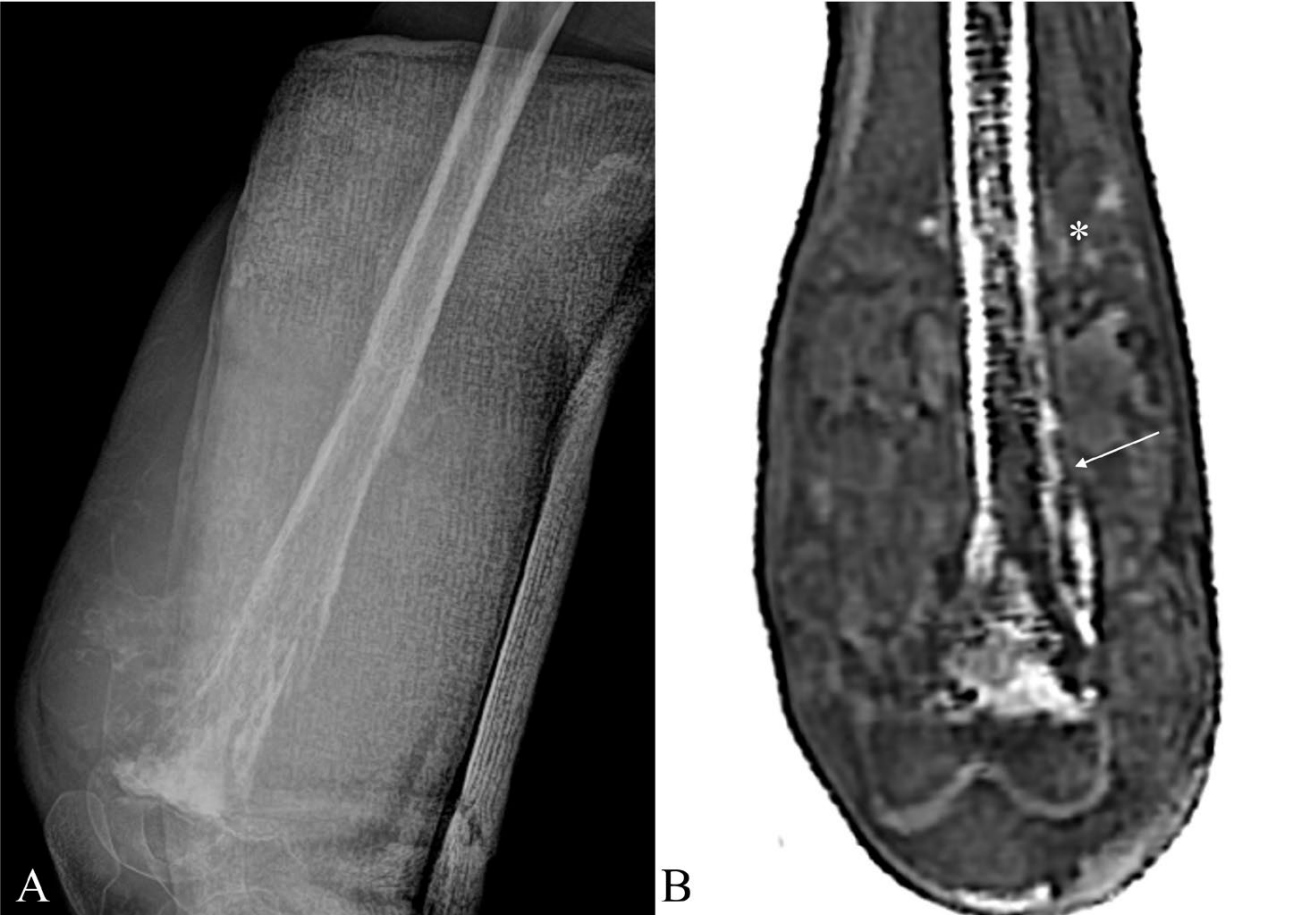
Anteroposterior radiograph (A) demonstrates an aggressive lesion involving the distal femoral diaphysis with associated periosteal reaction and soft‐tissue involvement. Corresponding zero echo time (ZTE) image (B) clearly depicts cortical breach (arrow) and adjacent soft‐tissue matrix mineralization (asterisk), further delineating the extent of disease.
